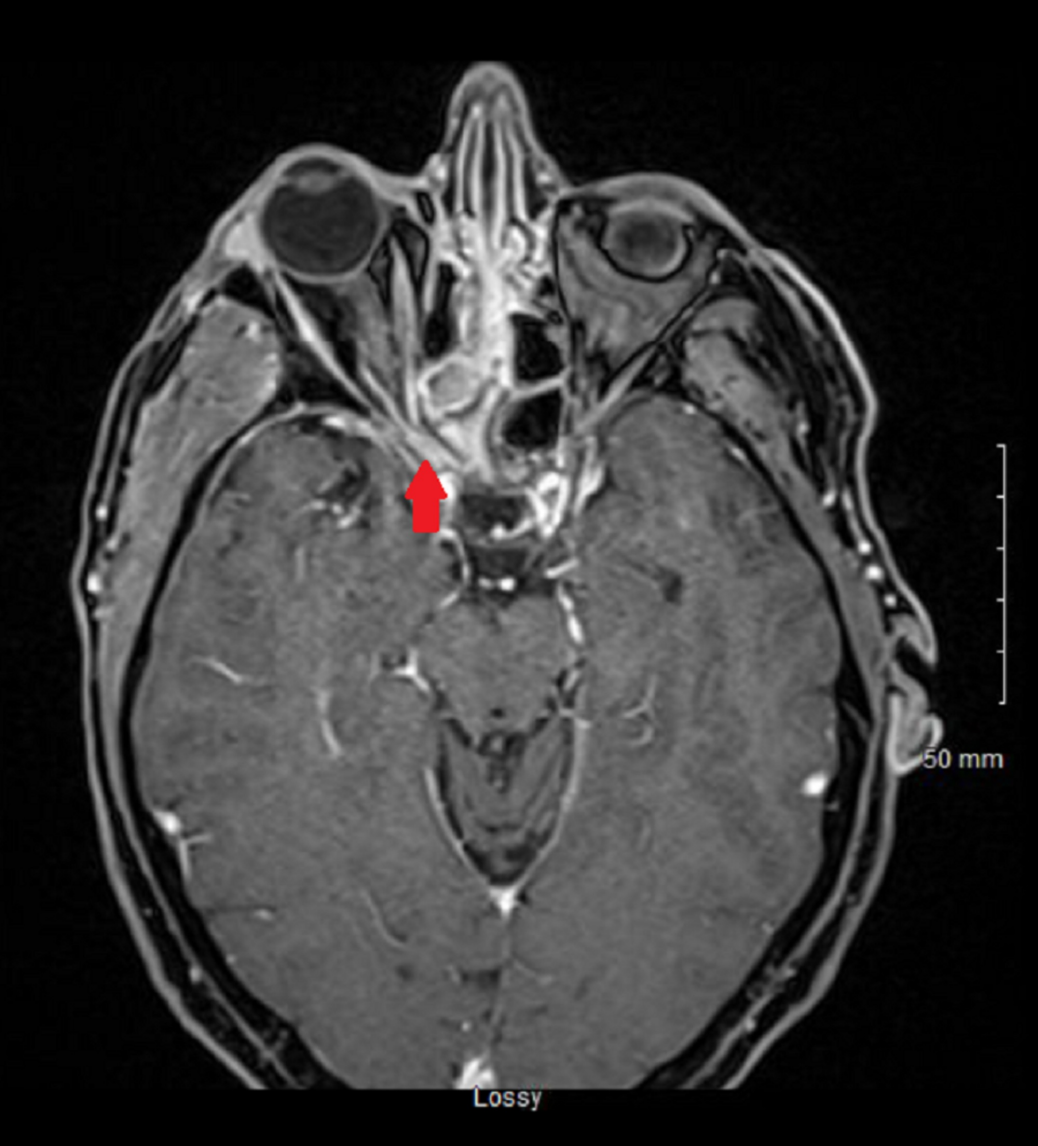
Magnetic resonance image of the axial section of the orbits and face Right eye proptosis, right-sided sinuses involvement, and compression of the right optic nerve (red arrow) can be seen.
